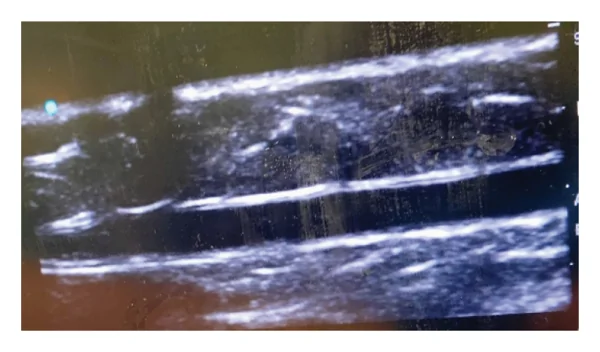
Ultrasound scan of the right great saphenous vein anterior to the medial malleolus.
